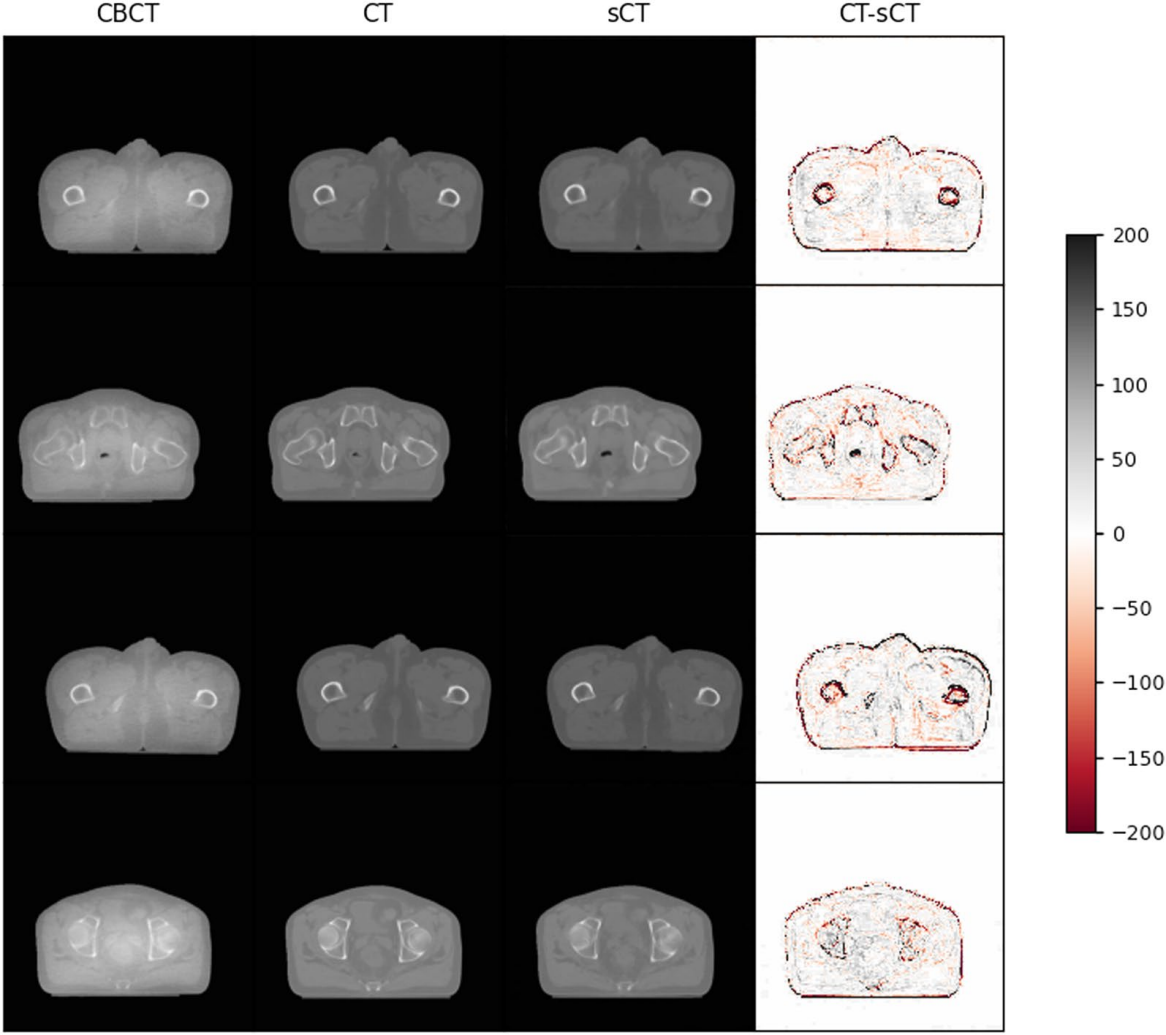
CBCT, CT and synthetic CT (sCT) images comparison from four different testing patients with different anatomical structures. The first column is CBCT images, the second column is real CT images, the third column is synthetic CT images and the last column is pixel-wise differences between real and synthetic CT images
